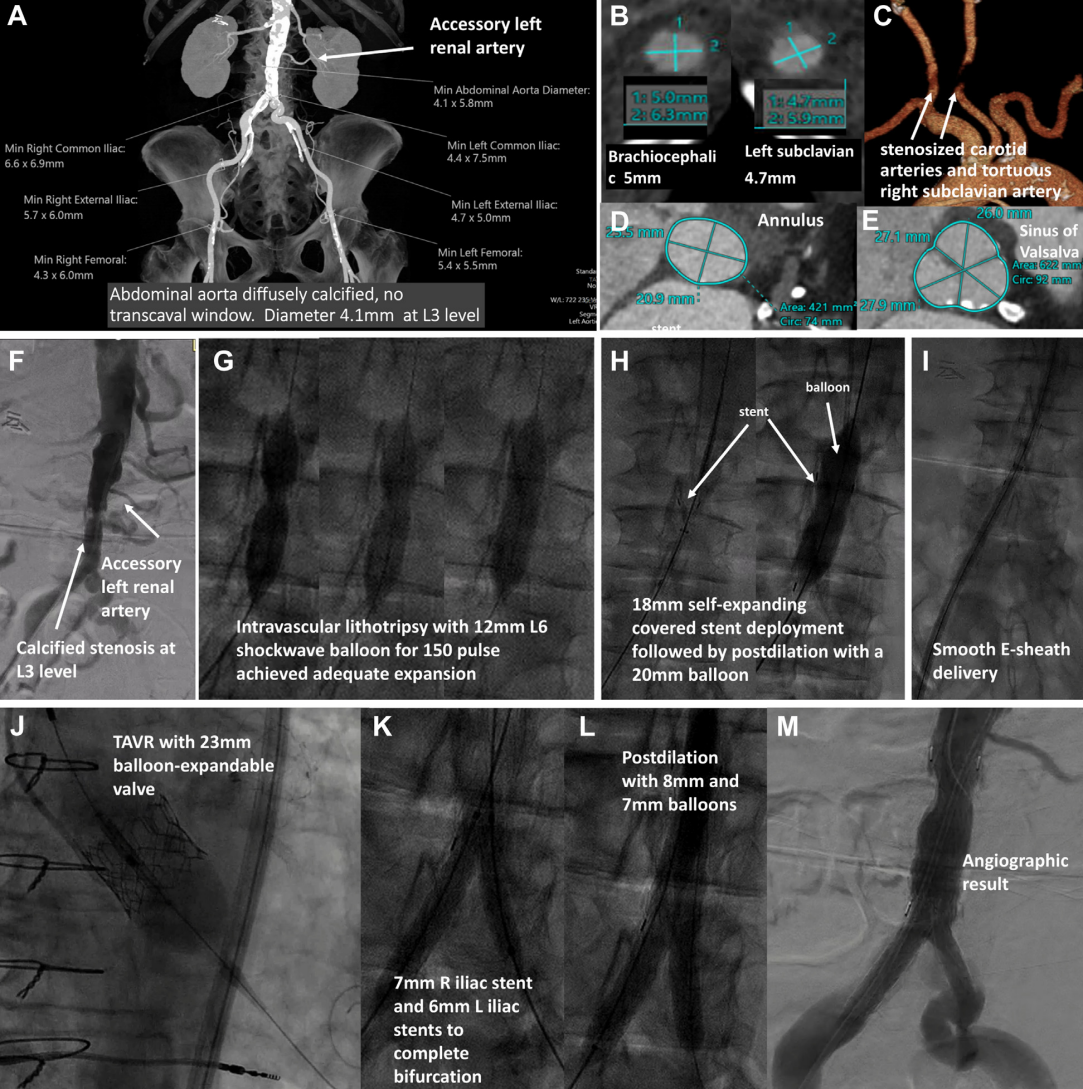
Workup and Procedure (A) Computed tomography of aorta and iliofemoral vessels, (B) brachiocephalic artery and right subclavian artery diameter, (C) bilateral carotid stenosis, (D and E) aortic annulus and sinus of Valsalva dimensions. (F) Angiogram showing abdominal aorta stenosis at L3 level and an accessory left renal artery. (G) Lithotripsy with a 12-mm L6 balloon for 150 pulses led to adequate expansion of the calcified stenosis. (H) Placement of a 18-mm covered stent followed by postdilation with a 20-mm balloon. (I) Smooth delivery of the E-sheath. (J) TAVR with 23-mm balloon expandable valve at +1.5 mL. (K and L) Completion of stenting iliac limbs and kissing balloon inflation. (M) Angiographic result. TAVR = transcatheter aortic valve replacement.
